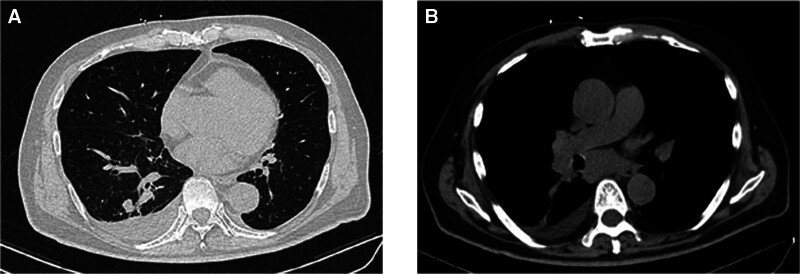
Chest CT scan before treatment. CT = computed tomography.
